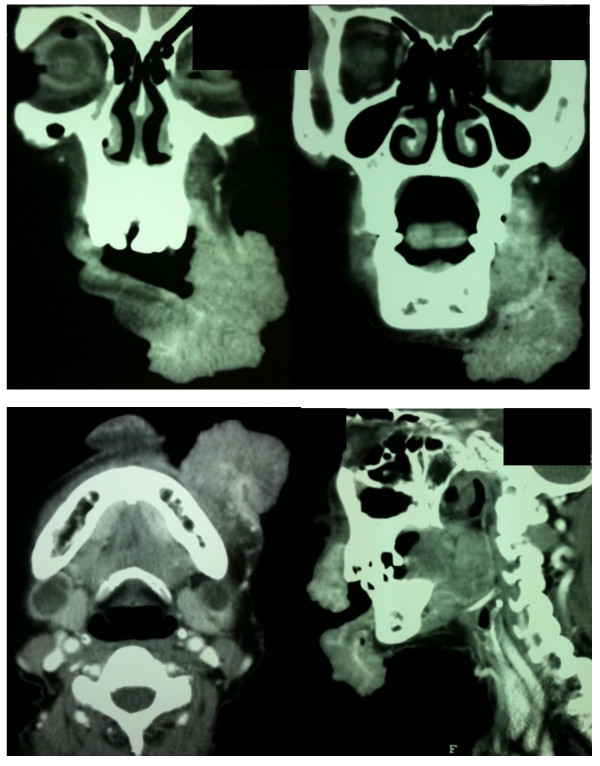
Head and neck computed tomography (CT) examination of patient. This CT scan showed irregular lobulated contour, heterogenous enhancing mass involving upper and lower lip (coronal view, axial view and sagittal view).
